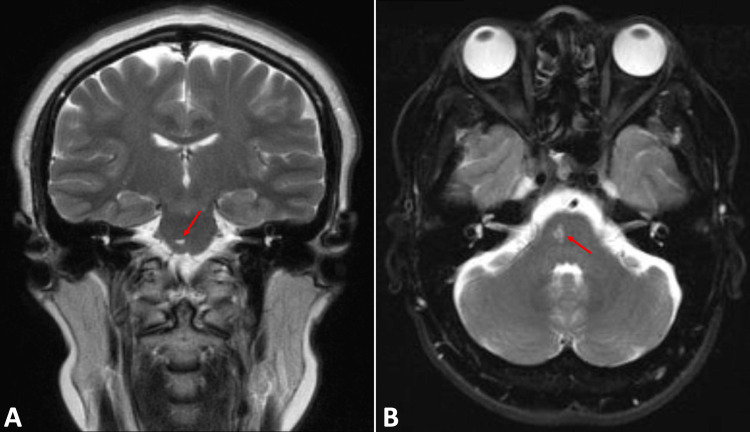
MRI of the brain without contrast demonstrating a small focal lobulated hyperintense T2 focus of the right pons measuring approximately 8 mm × 4 mm × 2 mm in coronal (A) and axial views (B). MRI = magnetic resonance imaging
